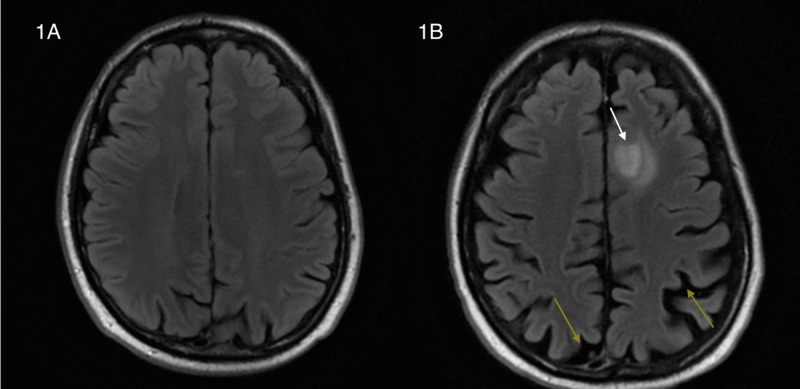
Axial FLAIR MRI of the brain. (A) Normal axial FLAIR MRI image two years prior. (B) Axial FLAIR MRI image demonstrating mostly bilateral parieto-occipital atrophy (yellow arrows) and large left frontal lobe plaque (white arrow). FLAIR, fluid-attenuated inversion recovery
